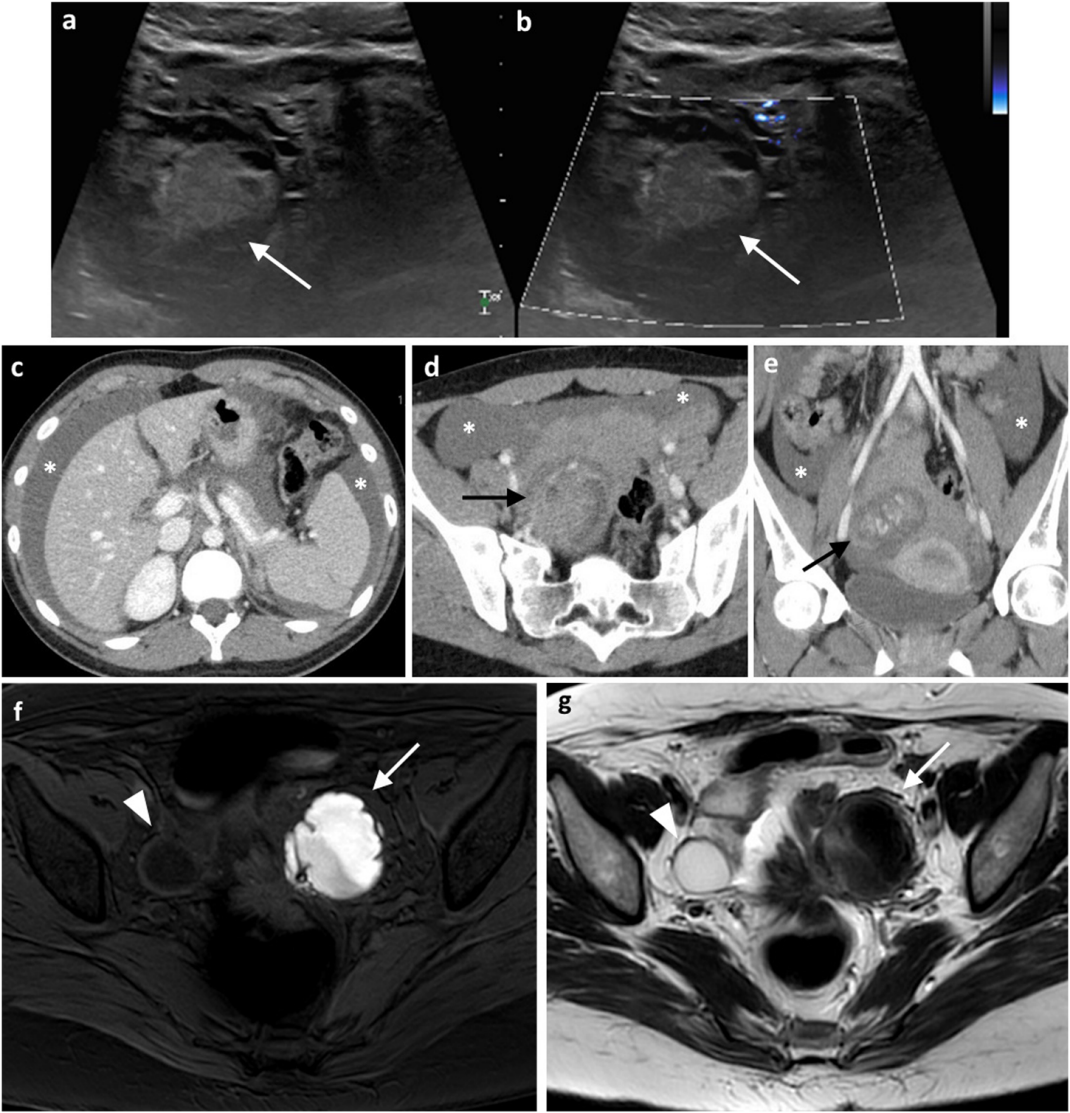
Complicated ovarian cyst in a young woman in her late teens presenting with acute pelvic pain, nausea and vomiting. a, b Transabdominal grey-scale and Doppler US shows a complex cystic mass with hyperechoic content (arrow) without vascularisation in Doppler images. c–e Portal venous phase axial and coronal CT images demonstrate a large amount of hemoperitoneum (asterisk) in the abdomen and pelvis. The images through the pelvis show a right para-uterine cystic mass (black arrows) with multiple hyperdense foci within related to active bleeding. Large hemoperitoneum with sentinel clot in the pelvis. Findings suspicious for ruptured ovarian cyst with active bleeding. Surgery confirmed ruptured corpus luteal cyst. f, g Endometrioma in a young woman with acute left quadrant pain. Axial T1-FS WI (f) shows a large left ovarian cyst with high signal intensity and a fluid-fluid level (arrow). In the axial T2 WI (g), the adnexal lesion shows marked signal loss due to cycling bleeding (shadowing sign). Note the right ovarian follicular cyst (arrowhead)
